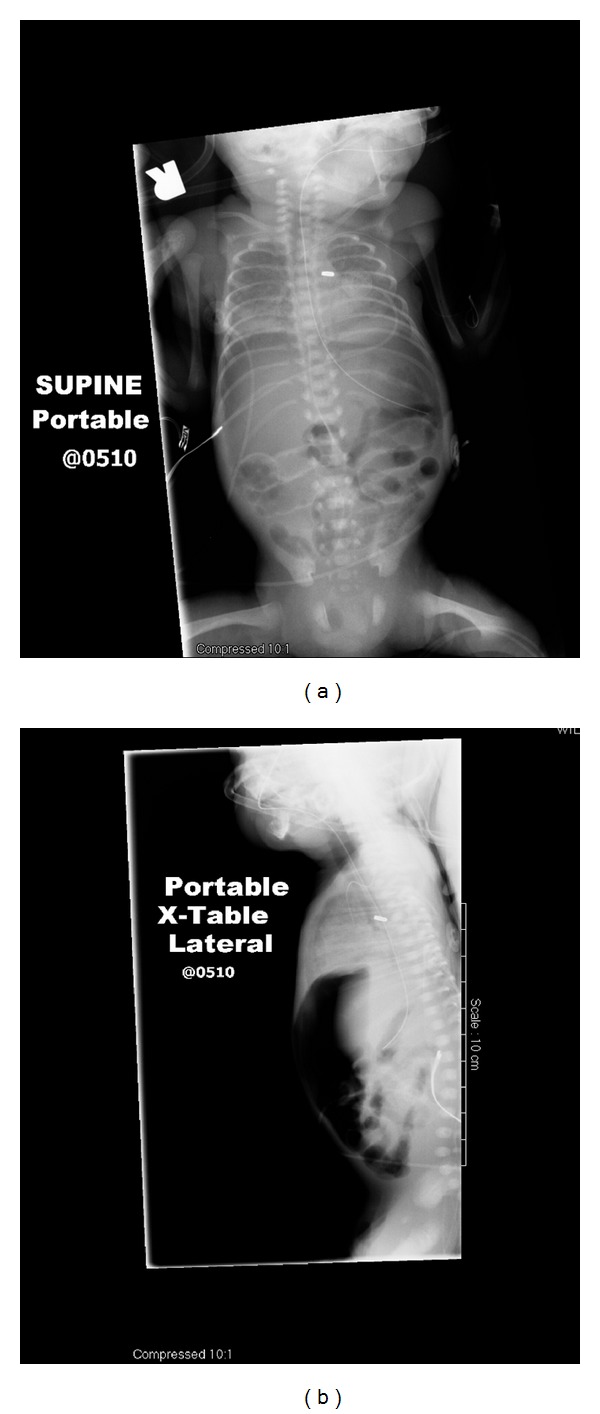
(a) AP abdominal X-ray on DOL 8 showing no portal venous gas or pneumatosis (b) cross table lateral X-ray on DOL 8 showing pneumoperitoneum.
